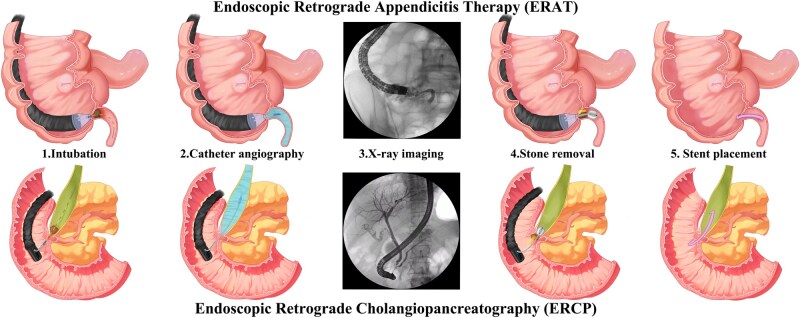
The similarities between ERAT and ERCP techniques (including intubation, catheter angiography, X-ray imaging, stone removal, and stent placement).
